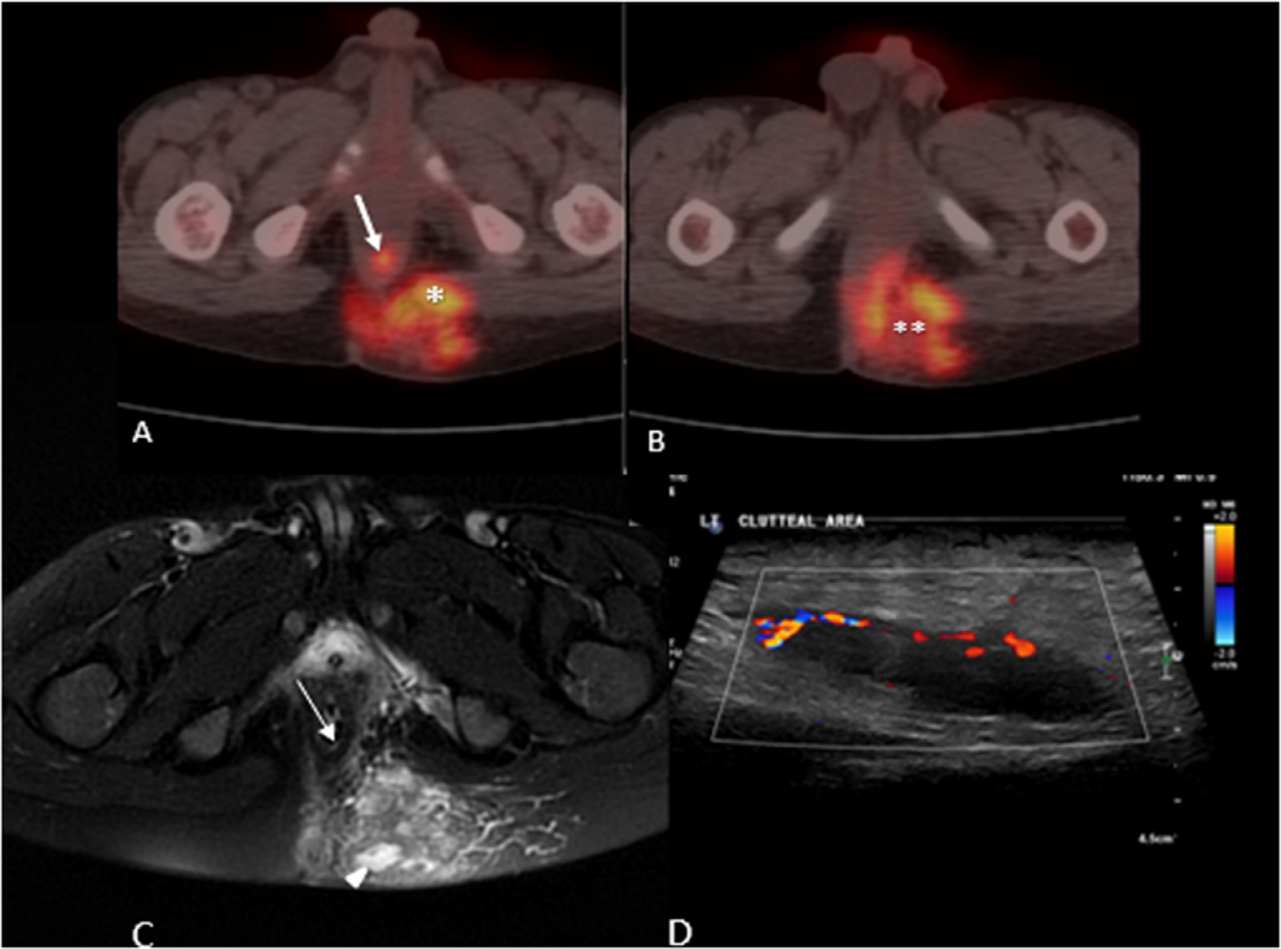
(A) 18 F-FDG PET/CT scan showing intersphincteric focal hypermetabolism suggestive of fistulous tract (White arrow) and hypermetabolism of left gluteal lesion (asterisk). (B) 18 F-FDG PET/CT scan showed mild central photopenia of the left gluteal lesion suggestive of necrosis/collection. (Double asterisks). (C) Axial MRI T2-FS showing heterogeneous signal intensity of the left gluteal lesion with focal high signal intensity suggestive of necrosis/collection (arrow head) and faintly visualized intersphicteric increased signal intensity suggestive of fistulous tract raising the suspicion of infectious/inflammatory process. (Thin white arrow). (D) Gluteal ultrasound showing anechoic structure with peripheral hypervascularity suggesting collection.
